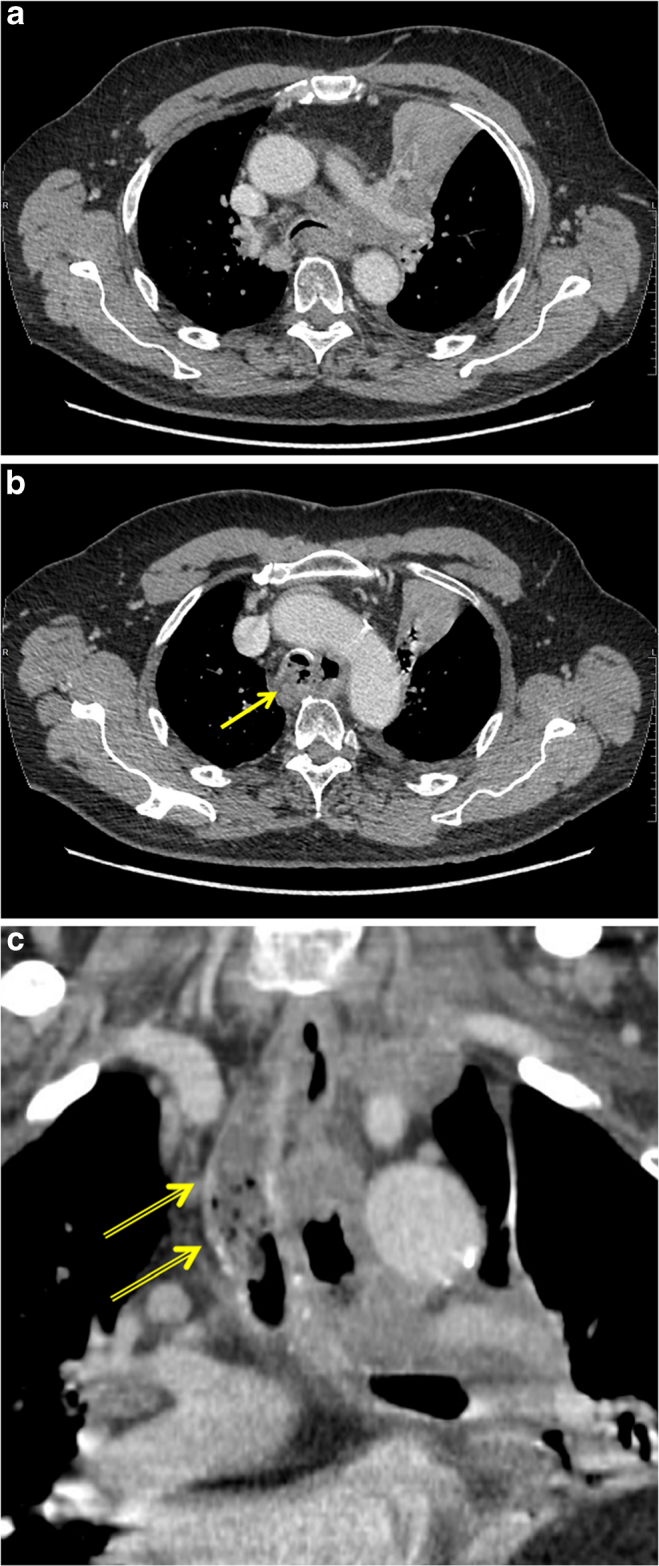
Tracheo-oesophageal fistula in a 60-year-old man with known stage IV non-small cell lung cancer who presented with a fever and had complaints of dysphagia. a Axial contrast-enhanced CT in mediastinal window setting shows a large left hilar mass encasing the left pulmonary artery with associated retro-obstructive atelectasis of the left upper lobe. Note mediastinal invasion extending to the trachea and oesophagus (*yellow arrow*). b Axial CT image at the level of the aortic arch reveals an extraluminal gas collection adjacent to both oesophagus and trachea. Both the walls of the oesophagus and trachea are thickened at this level with blurring and fatty infiltration of the surrounding fat planes. c Reformatted image in the coronal plane nicely depicts the presence of fluid, debris and air-bubbles in the trachea (*double yellow arrows*). The combination of findings is indicative of a tracheo-oesophageal fistula
